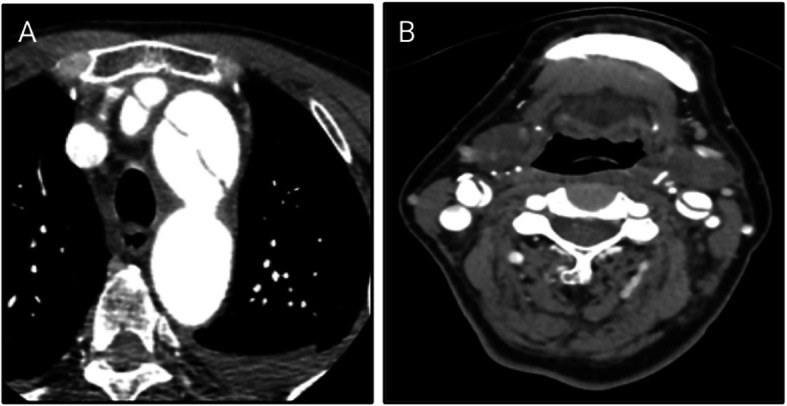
CT angiography (CTA) images of the patient showed dissection of the ascending aorta, aortic arch and bilateral common carotid arteries
